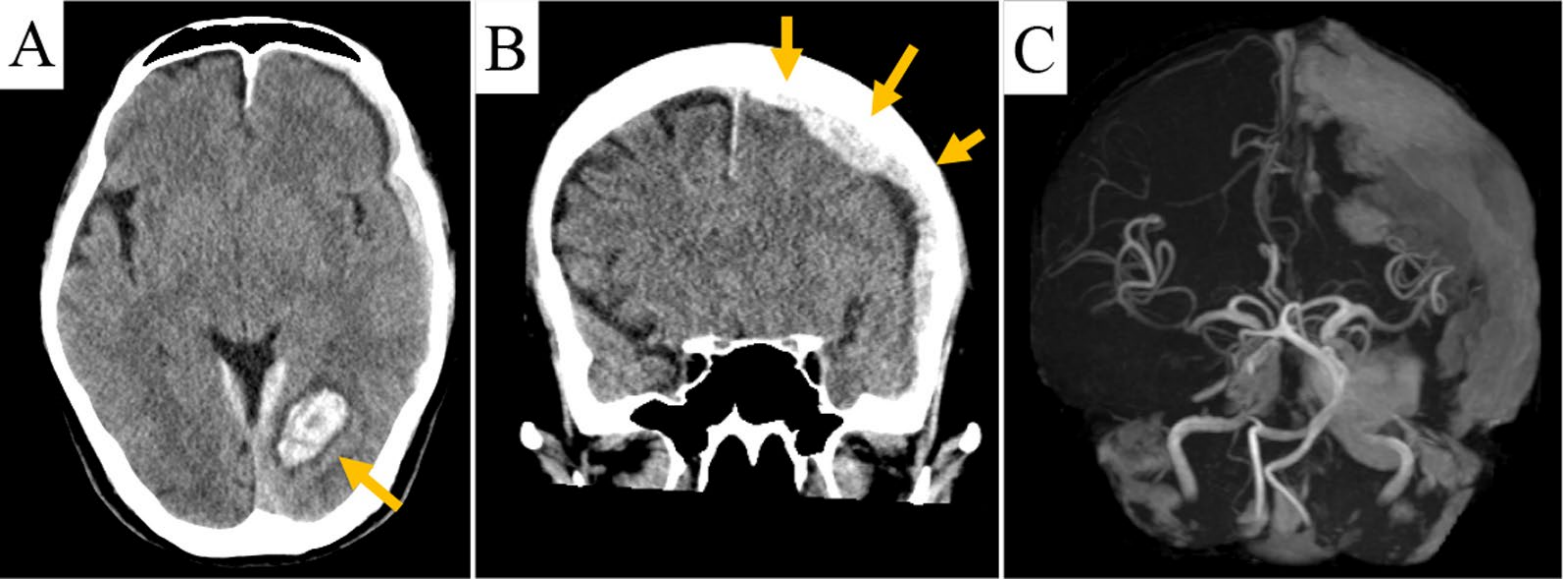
A, B Computed tomography showed a left acute subdural hematoma and hemorrhage, and a left subcortical occipital lobe hemorrhage. C Magnetic resonance angiography did not reveal any blockage of the major vessels, and no mycotic aneurysms were observed
